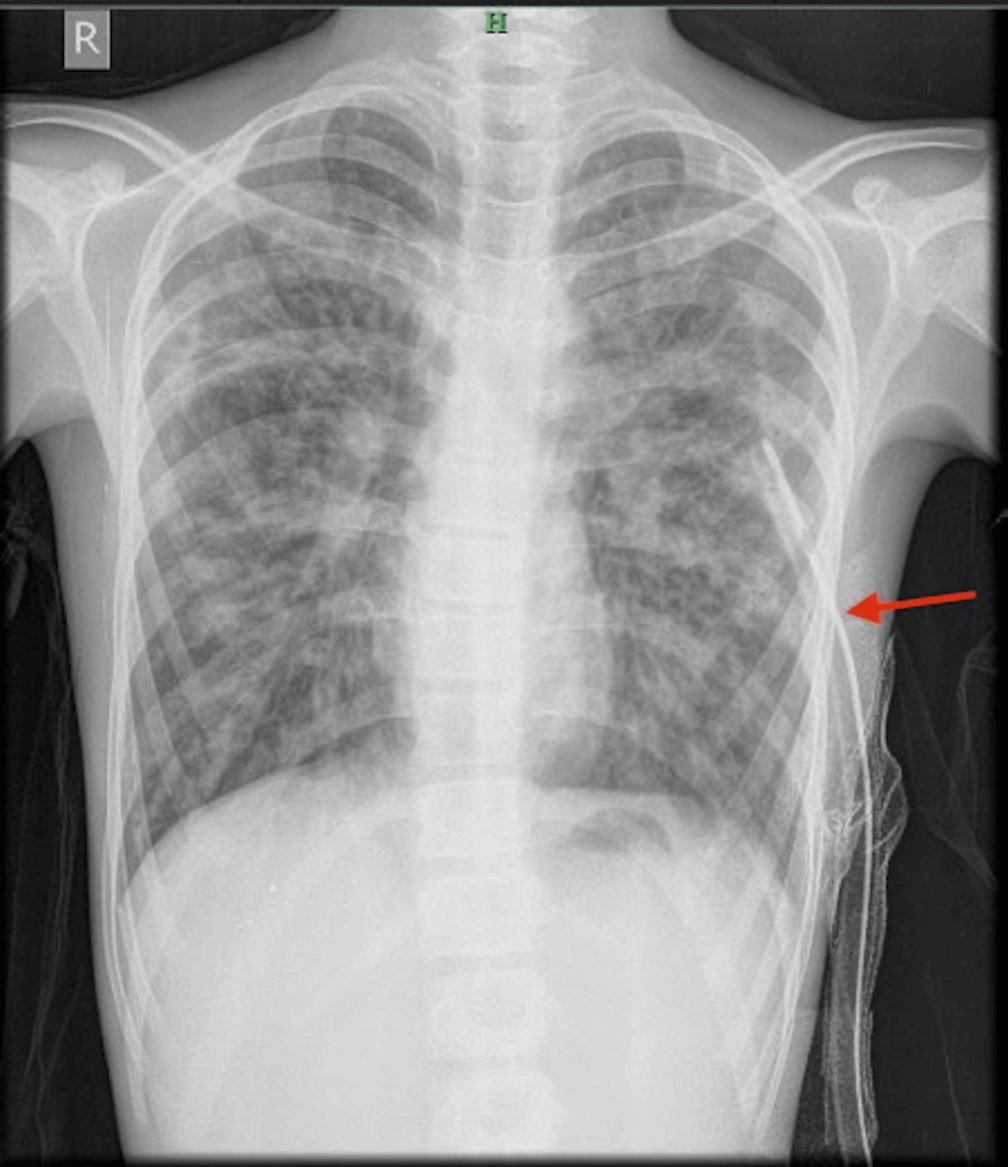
Chest X-ray with intercostal tube in situ Red arrow: intercostal tube
